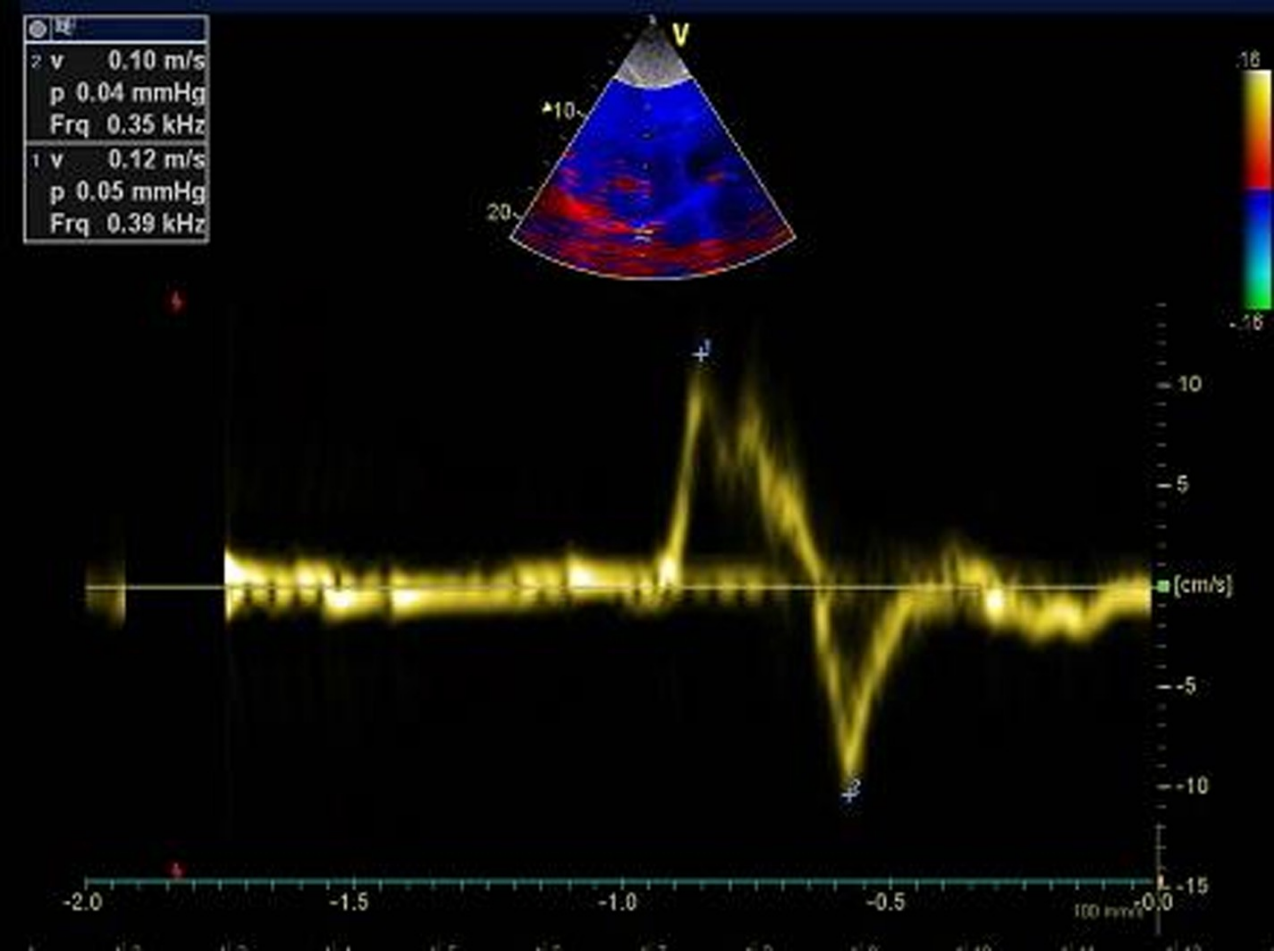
Sniff right hemidiaphragm tissue Doppler imaging peak velocity (arrow) in a volunteer (peak velocity = 12 cm/s).
